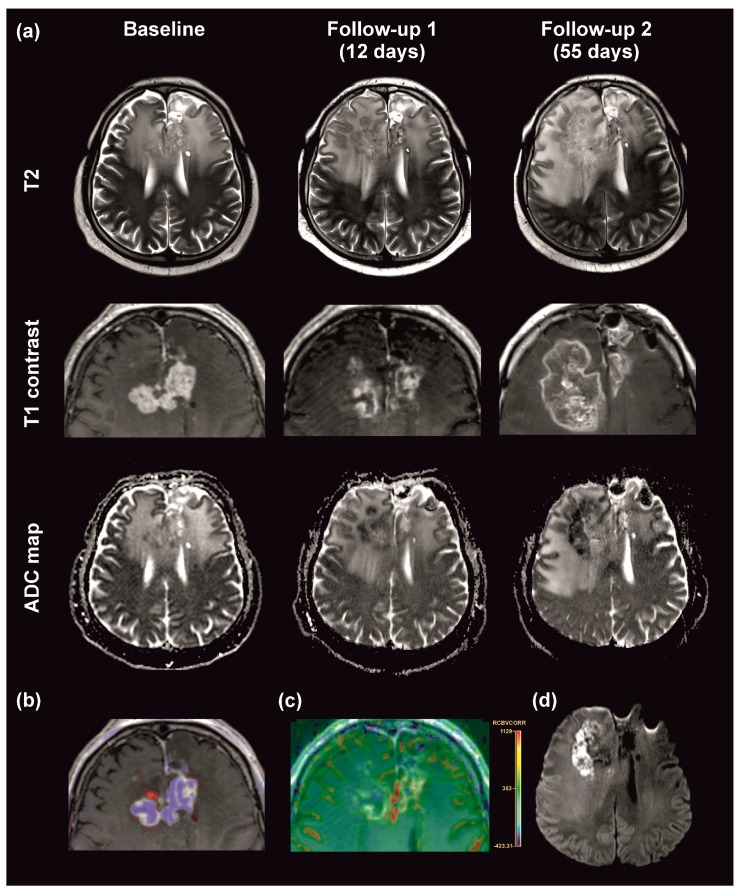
Magnetic resonance imaging (MRI) alterations in a regorafenib-induced T2-dominant progression pattern. (a) MRI scans were performed at baseline and the indicated intervals after initiation of REG therapy. T2 weighted images (T2, upper row), contrast enhanced T1 weighted images (T1 contrast, middle row), and apparent diffusion coefficients (ADC) maps (lower row) are depicted. “T2-dominant” progression is characterized by an overall decrease of contrast-enhancement and simultaneous increase in T2 hypointense tumor areas in the right frontal lobe. (b) Comparison of T1 contrast images at baseline and after 12 days. Areas of decreasing signal intensity are segmented in blue, areas of increasing intensity are segmented in red. (c) Color-coded perfusion map for leakage corrected cerebral blood volume (CBV) showing patchy, red areas with markedly increased CBV within the tumor outside of areas with reduced ADC. (d) Diffusion-weighted b 1000 image corresponding to corresponding ADC map at 55 days.
